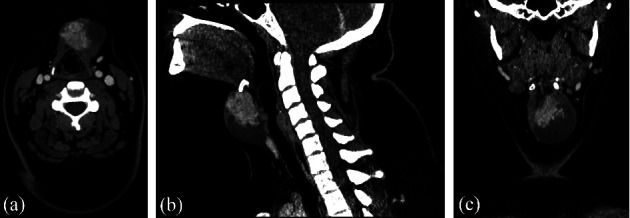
Contrast-enhanced CT showing a sizeable (4.38 × 4.26 cm) well-circumscribed heterogeneous mass anteroinferior to the hyoid bone in axial (a), sagittal (b), and coronal (c) planes.
